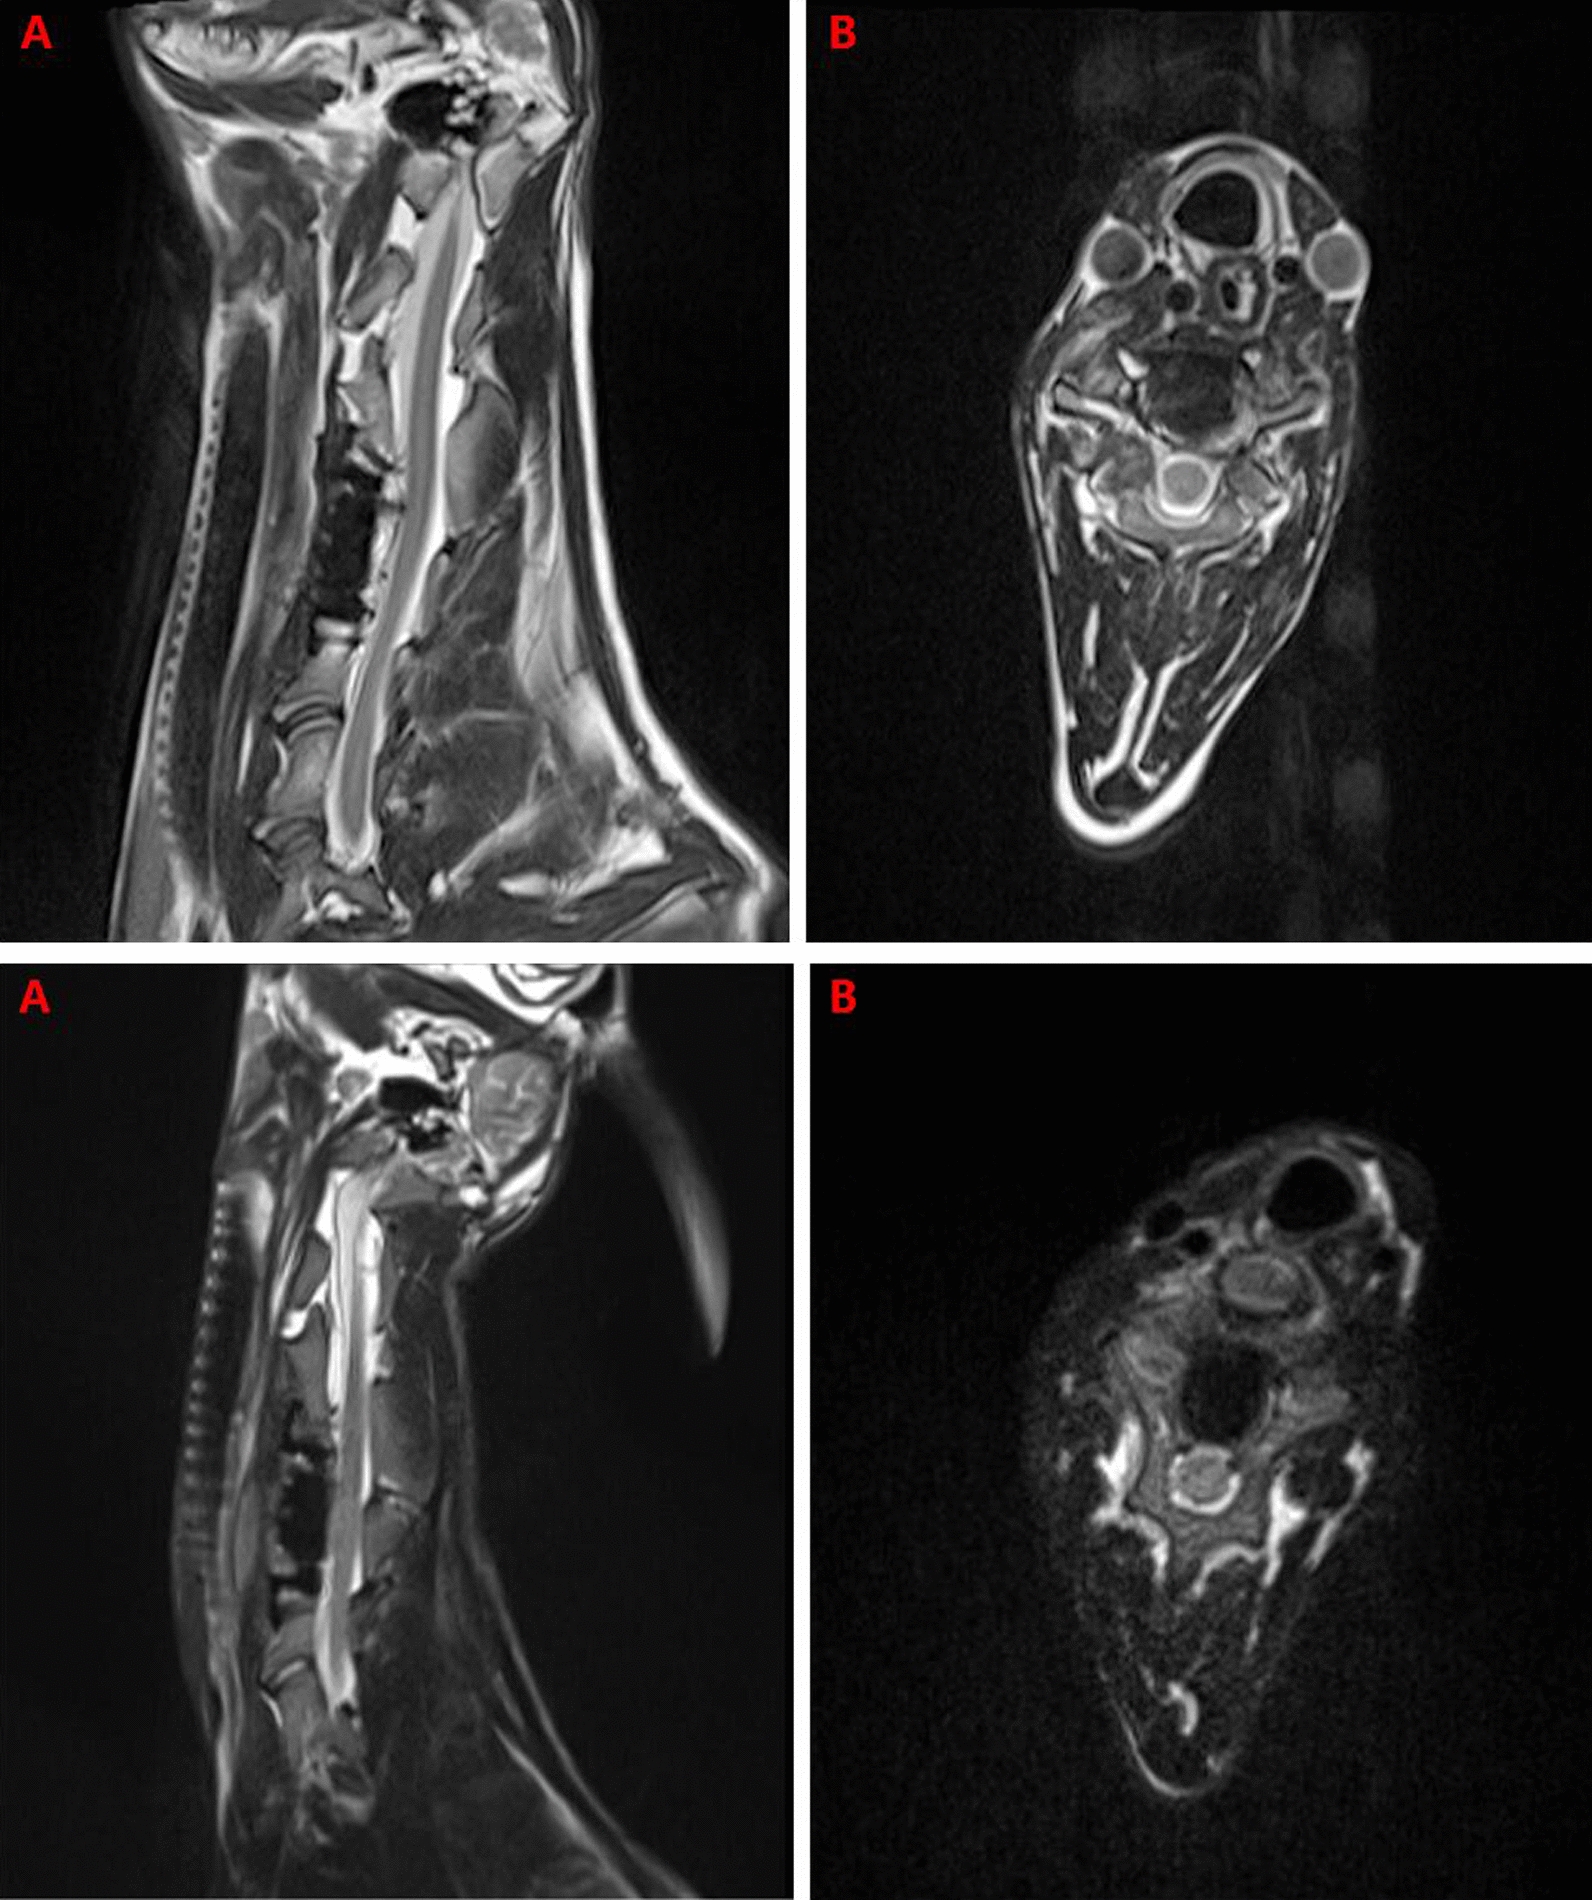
1 MRI 3 months after surgery. A, Sagittal view. B, Axial view. 2 MRI 6 months after surgery. A, Sagittal view. B, Axial view
